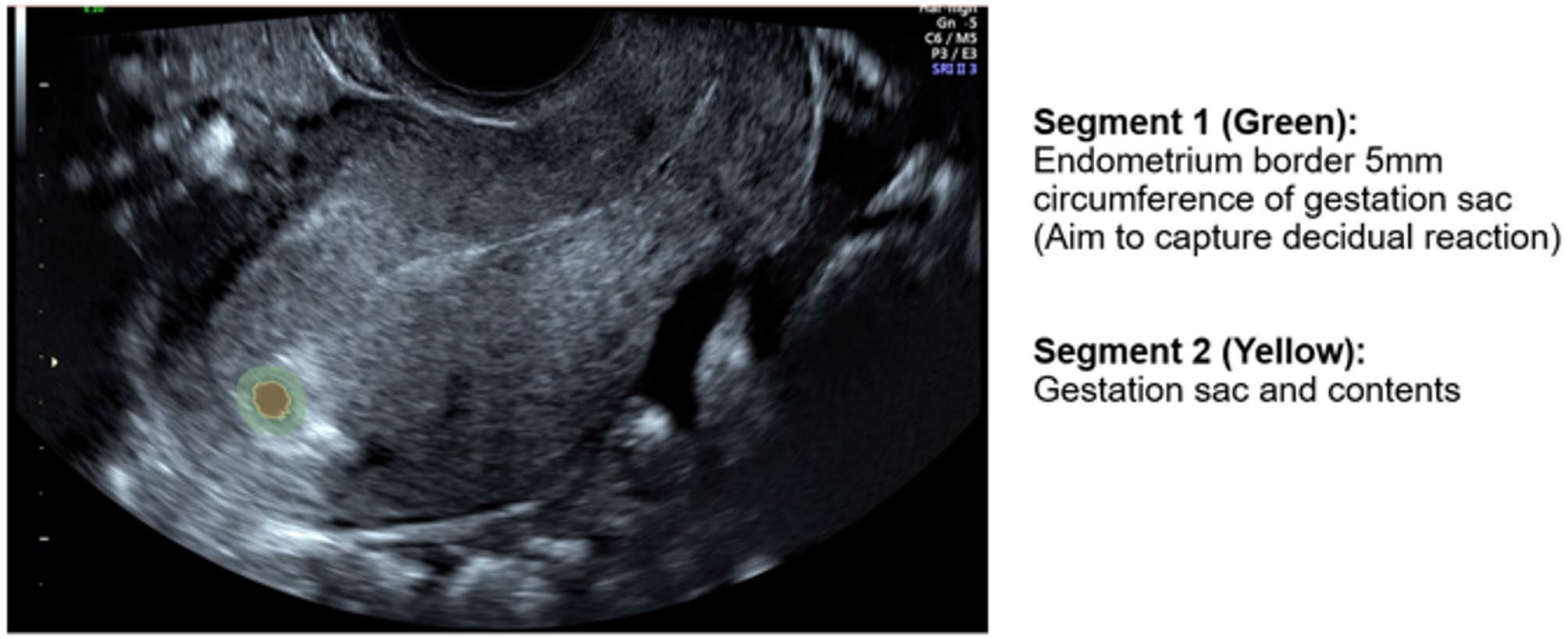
Example of Ultrasound Image with the two regions of interest segmented.
